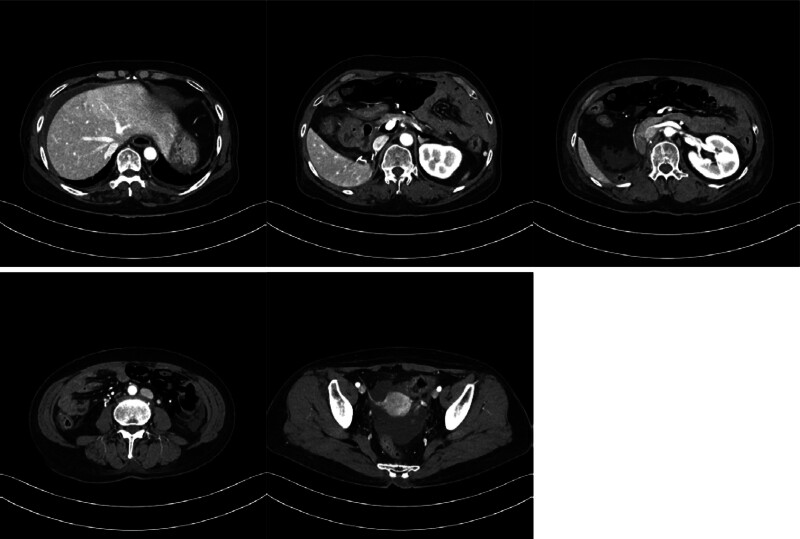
Abdominopelvic CT performed on the day of admission.
